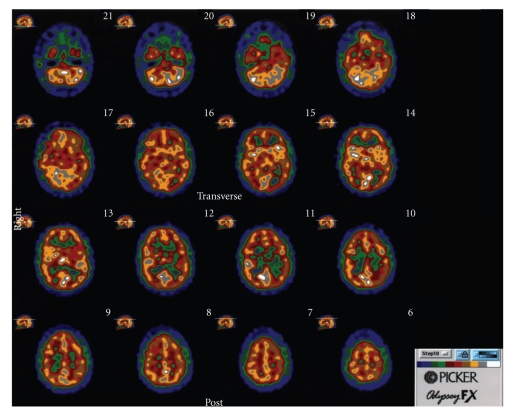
Age-22 SPECT scan showing prominent and heterogeneous hypoperfusion. One color step equals a 10% change in perfusion. The highest perfusion is indicated by white. See text for acquisition conditions and Table 4 for results of semiquantitative analysis.
